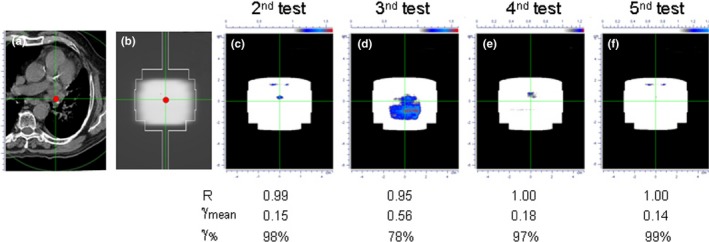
Detection of a poor treatment reproducibility in a patient treated using the ABC spirometer. From left to right, (a) the CT scan at isocenter level; (b) the reference integrated EPID image; (c–f) the IVD tests with a relevant discrepancy provided by *γ*‐analysis in figure (d).
